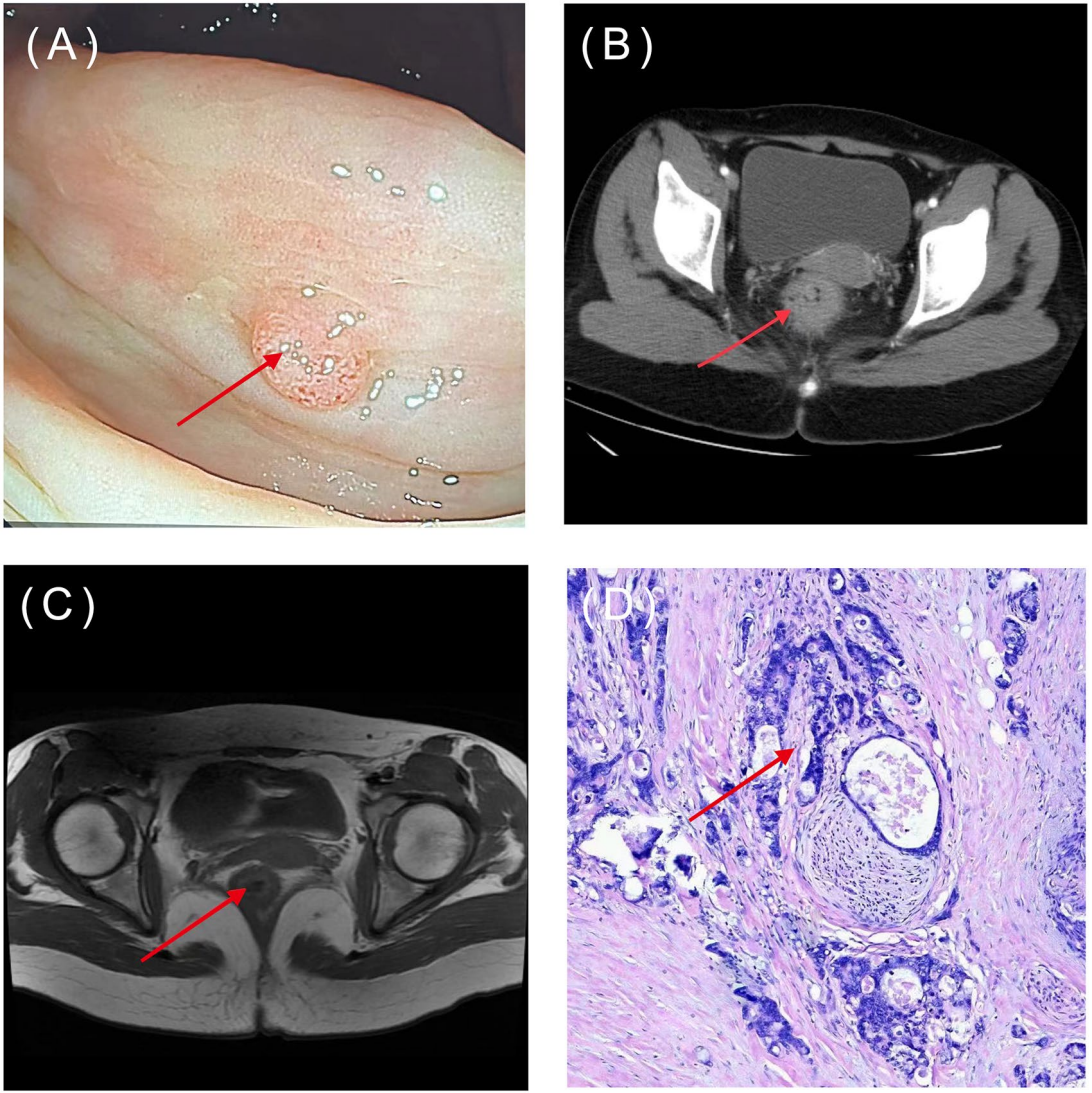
Common types of CRC diagnostic images: (A) Endoscopy; (B) CT; (C) MRI; (D) pathology image (HE×100). The arrow indicates the location of the lesions. (Image source: The First Affiliated Hospital of Dalian Medical University.)
